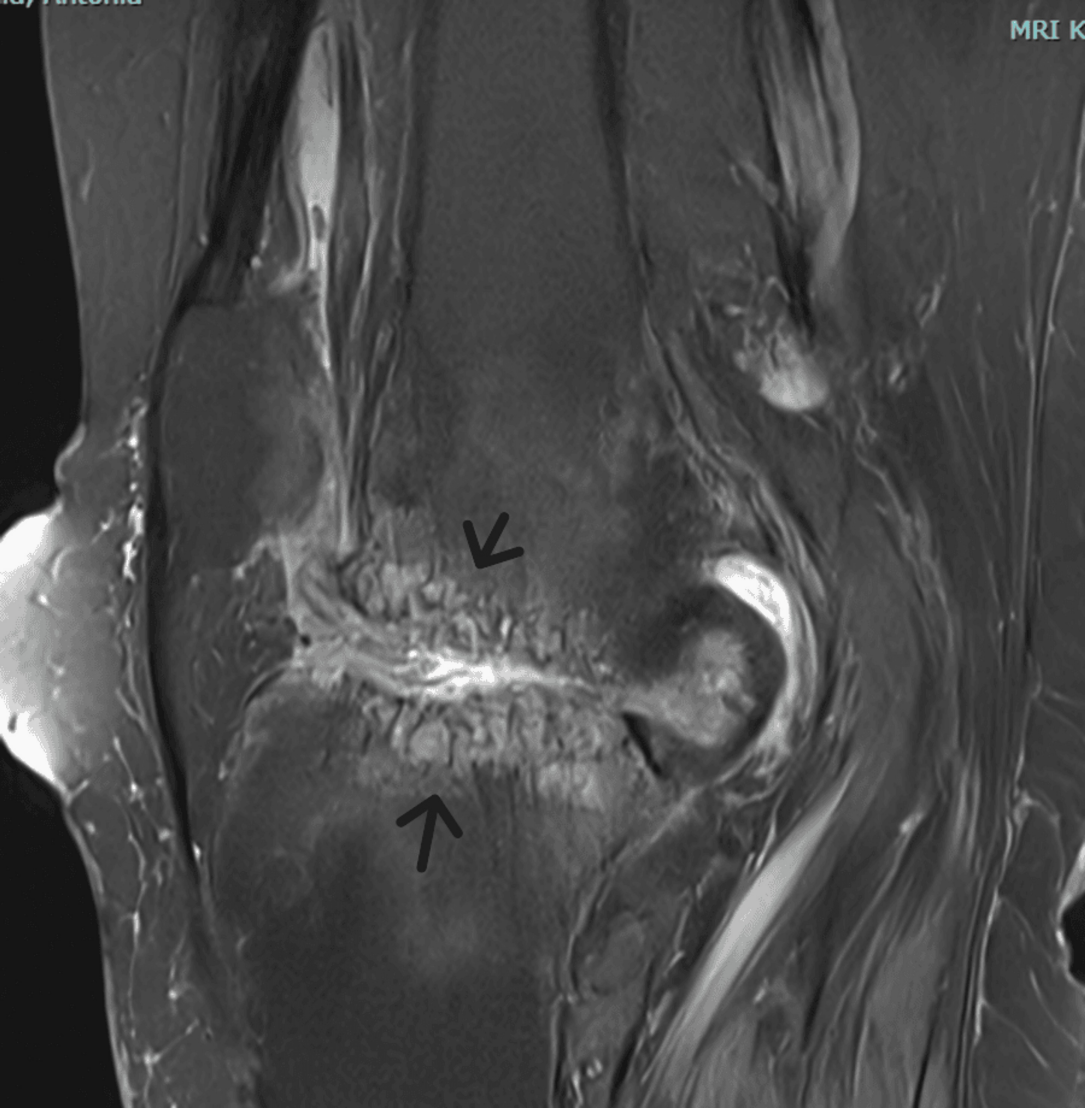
Τ2 weighted image. MRI of the right knee, sagittal view. Arrows pointing towards evidence of joint line erosion.
